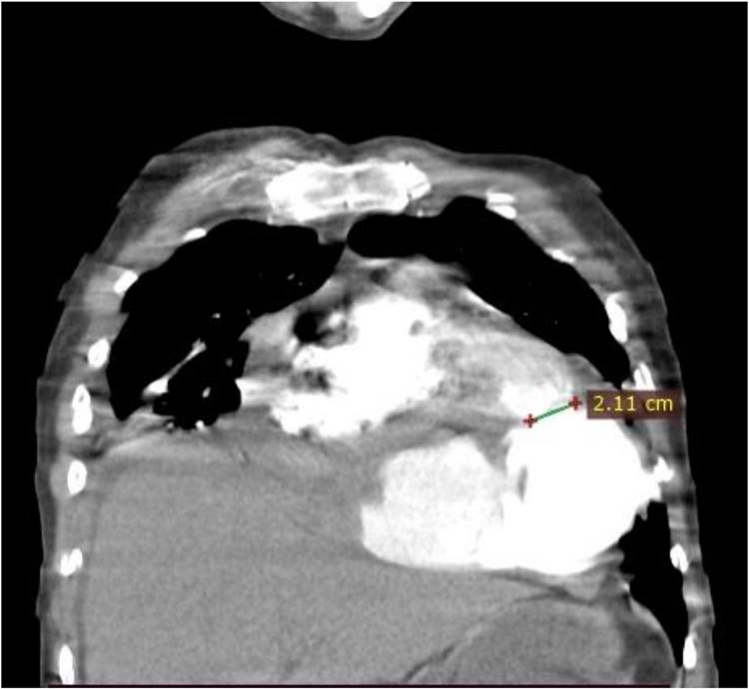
Coronal CT scan image. The figure shows a 2.1 cm communication between left ventricle and pseudoaneurysm. The heart is pushed upwards.
